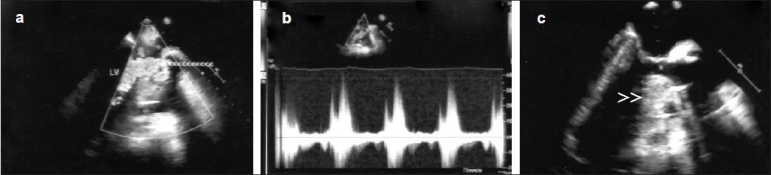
Intraoperative transesophageal echocardiogram following AVR showing turbulent abnormal flow in the LVOT (a, arrow heads) with a dynamic peak gradient of 58 mmHg (b) and a small left ventricle with thick basal sigmoid shaped septum (c, arrow heads) causing narrow LVOT; LV, left ventricle
